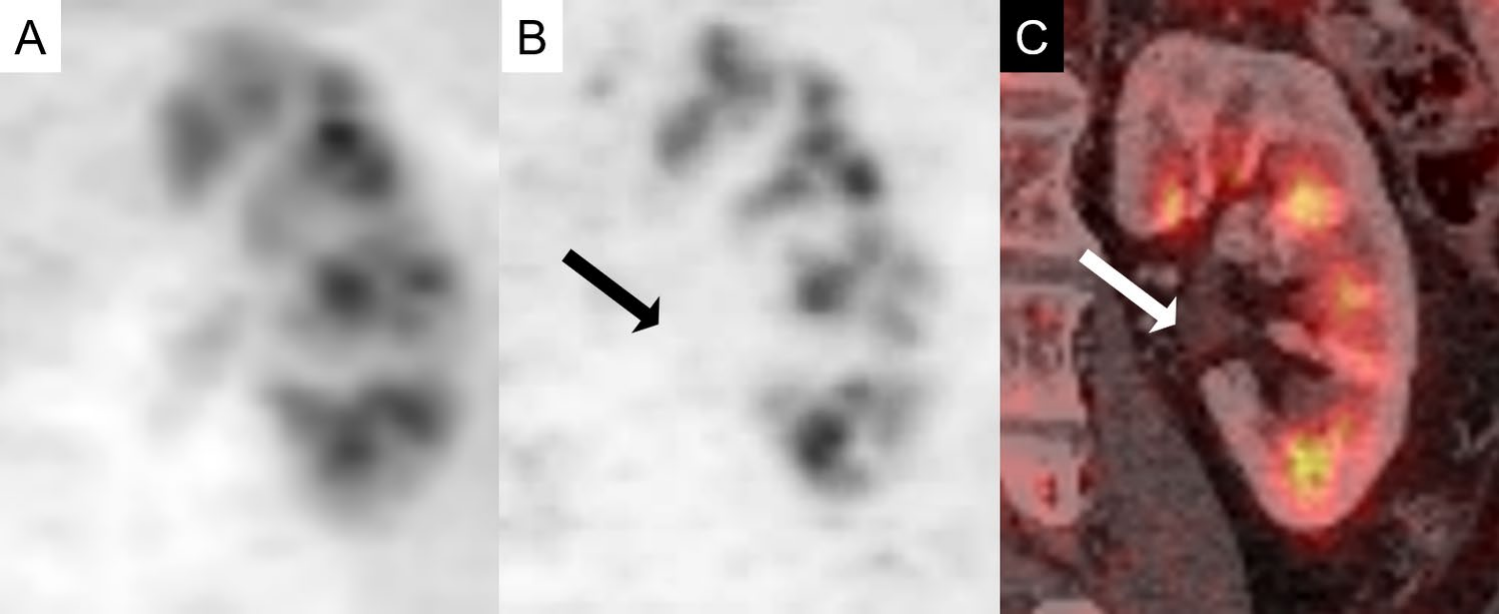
Physiological ^18^F-fluorodeoxyglucose (FDG) uptake of the kidneys. A Coronal FDG-positron emission tomography (PET) shows higher physiological accumulation in the renal medulla than in the cortex. B Coronal FDG-PET and C its fused image with contrast enhanced CT after administration of diuretics. Urinary FDG concentrations excreted in the renal pelvis (arrows) are decreased, as is accumulation in the renal cortex. Accumulation in the renal medulla is still present
